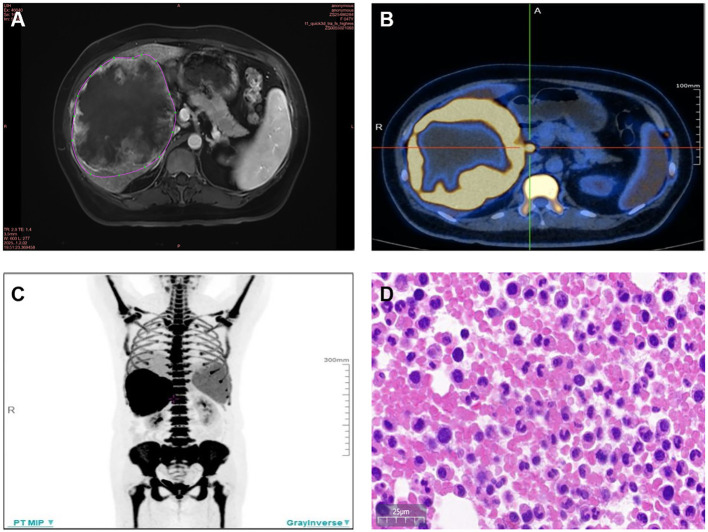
Diagnostic workup showing the hepatic lesion and the misleading hematologic findings. (A) Contrast-enhanced abdominal magnetic resonance imaging shows a large heterogeneous mass in the right anterior liver, with internal necrosis and involvement of adjacent hepatic vessels. (B) ^18F-FDG PET/CT fusion imaging demonstrates intense hypermetabolism of the hepatic mass with a central photopenic necrotic area. (C) Maximum intensity projection image from ^18F-FDG PET/CT shows diffuse bone marrow hypermetabolism and increased splenic uptake, initially raising concern for a hematologic malignancy. (D) Bone marrow aspirate smear shows marked granulocytic hyperplasia with left shift and no excess blasts, favoring a leukemoid reaction rather than a primary hematologic malignancy.
